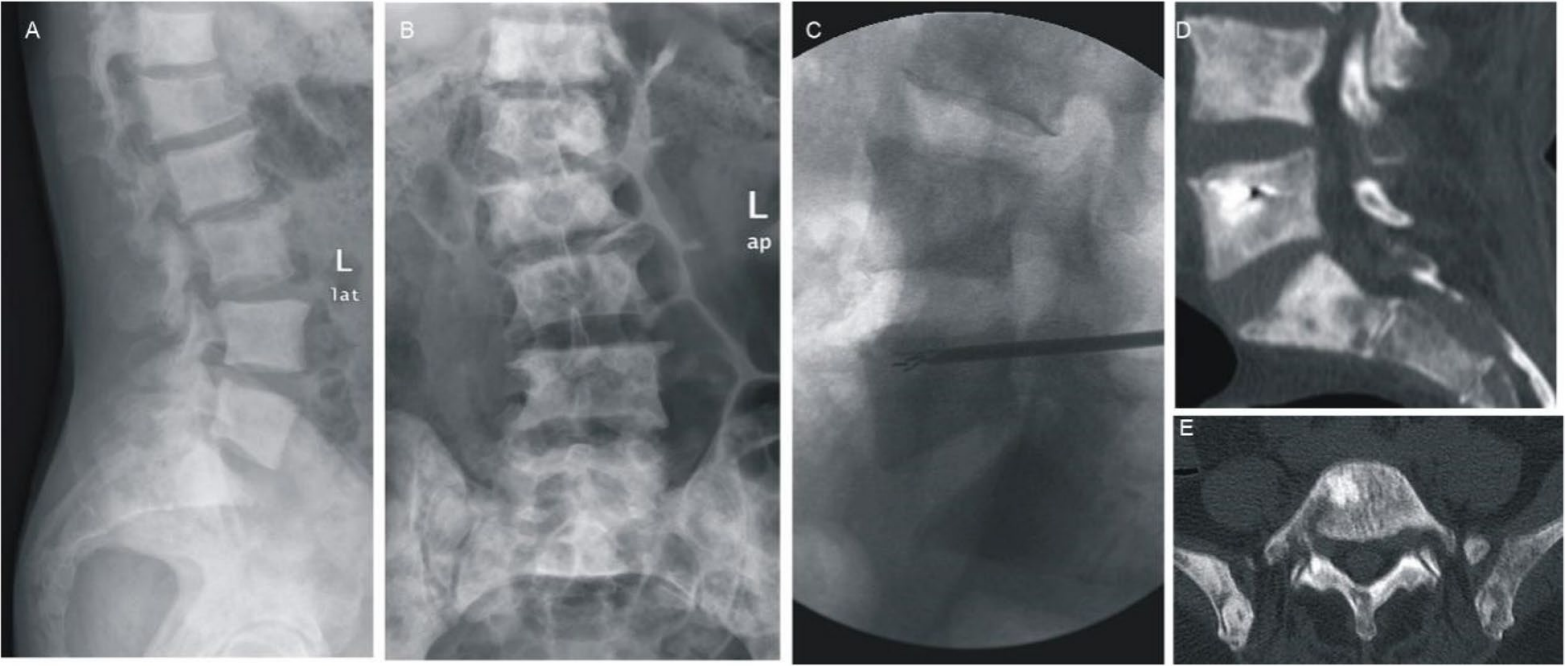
Male patient, 59 years old, well-differentiated prostate cancer after combined therapy for 1 year, back pain 8 months, preoperative VAS score of 8.5. A, B Preoperative DR shows multiple bone destruction lumbosacral, and pathological fractures. C L5PVP combined with ^125^I seed implantation. D, E Postoperative CT showed bone cement and ^125^I particle distribution in the lesion area, without leakage of bone cement. Postoperative VAS score 2.3 points, significant pain relief
